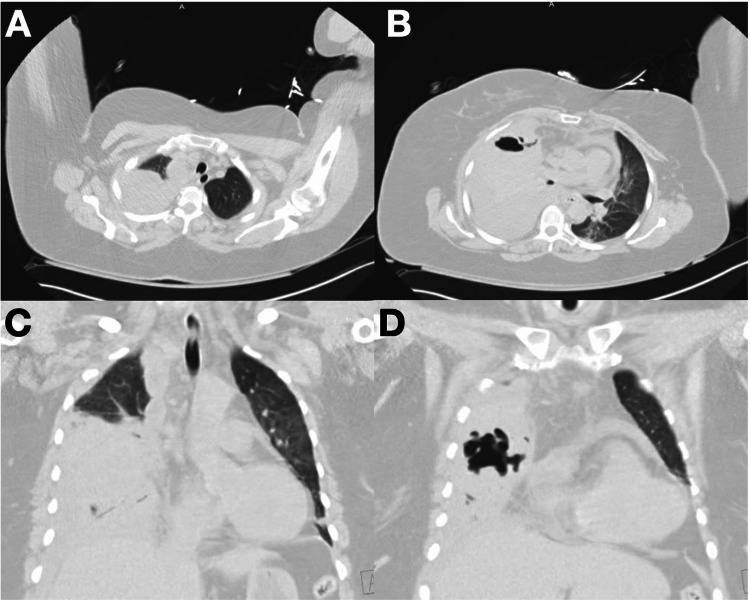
Computed tomography of the chest without contrast performed on the day of admission. (A and B) Computed tomography of the chest without contrast axial view on the day of admission demonstrated near-complete opacification of the right lung and a small amount of aerated parenchyma in the right upper lobe. (C and D) Computed tomography of the chest coronal view without contrast on the day of admission. There is a cavitation with a probable fluid level in the right middle lobe. Also showing moderate-to-large, low-density right pleural effusion.
